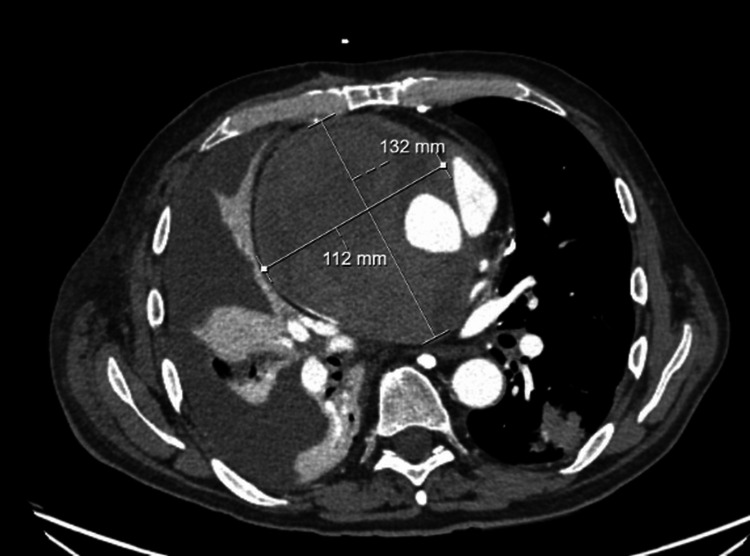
CT aorta on presentation A 13.2 × 11.2 × 11.5 cm graft leak into a partially thrombosed pseudoaneurysm/hematoma of the ascending aorta, with compression of the right upper lobe, right pulmonary artery, proximal right coronary artery, and superior vena cava.
